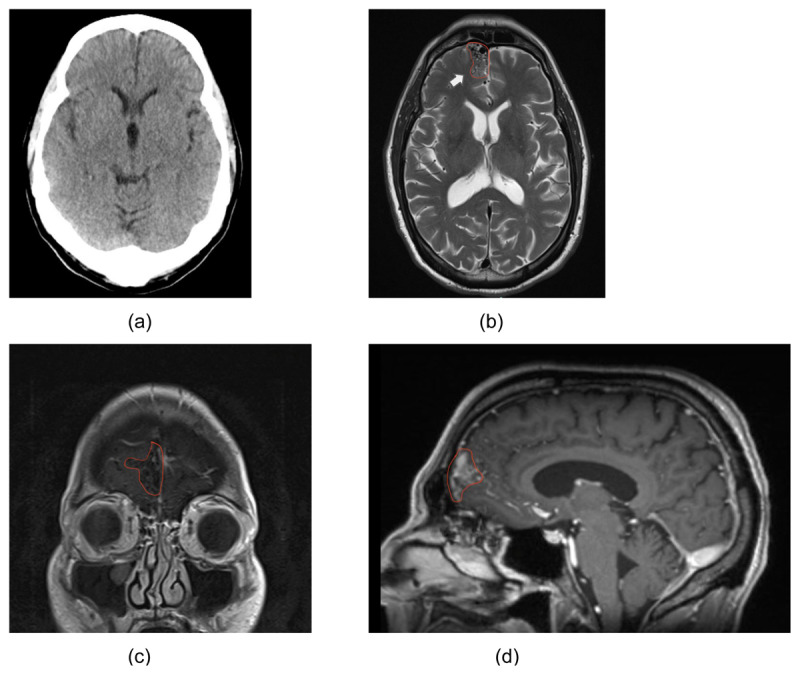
(a) CT-scan originally done during the work-up process. (b) T2-weighted Axial MRI demonstrating the AVM at the right frontal lobe. (c) T1-weighted sagittal MRI of the AVM at the right frontal lobe. (d) T1-weighed coronal MRI of the AVM at the right frontal lobe.
